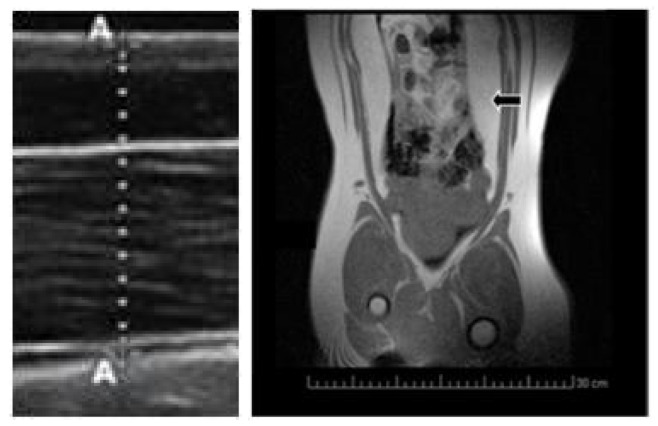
Imaging of subcutaneous fat depth by ultrasonography (left hand) and visceral fat depots by magnetic resonance imaging (MRI; right hand; black arrow).
